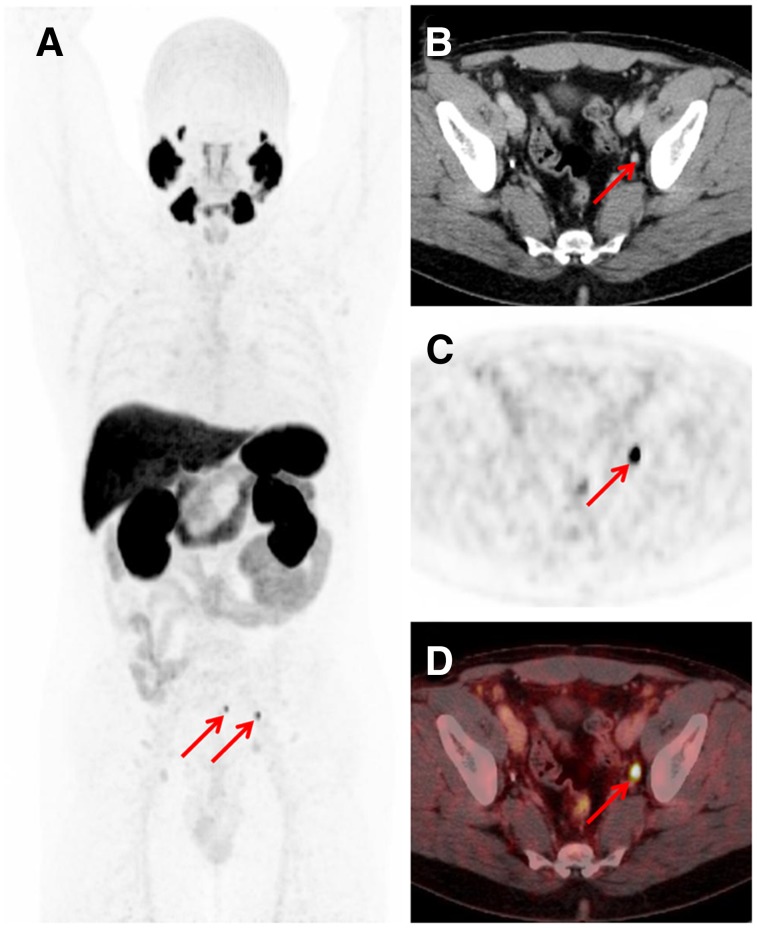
Images from 57-y-old patient after radical prostatectomy (2010; Gleason score of 8; pT3b; pN0), after salvage radiation therapy to prostate bed, and with PSA level rising to 0.43 ng/mL (August 2017). (A) Maximum-intensity projection of ^18^F-PSMA-1007 PET shows 2 intense tracer-associated lesions in left pelvic region (arrows). (B–D) Transaxial PET (C) and fused PET/CT (D) images show high ^18^F-PSMA-1007 uptake in subcentimeter (7-mm) lesions (arrows), as determined by corresponding CT (B). Subsequent radioguided salvage surgery proved malignant nature of lesions.
